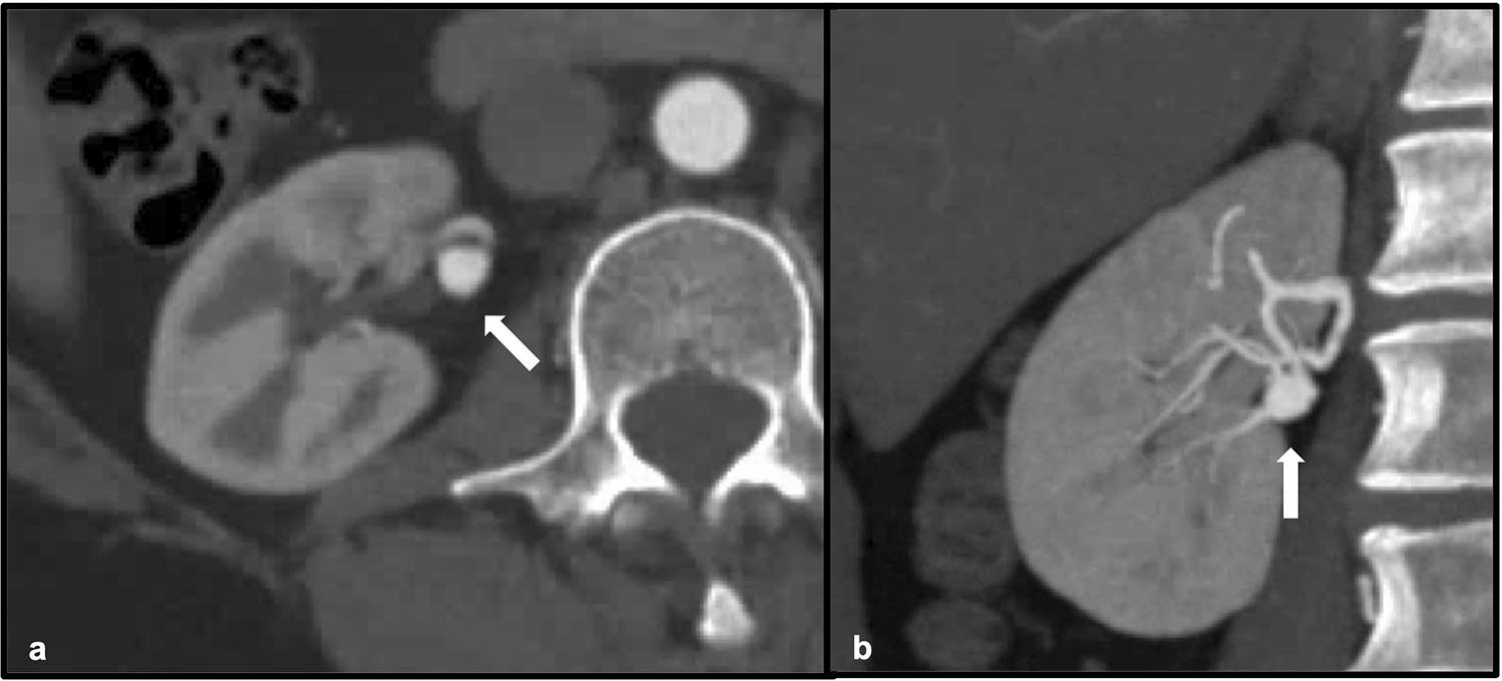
58-year-old female with a history of PAN and report of incidentally noted visceral aneurysms on outside imaging. Axial (a) and coronal MIP (b) contrast-enhanced CT images demonstrate aneurysmal dilation of a branch of the right renal artery
